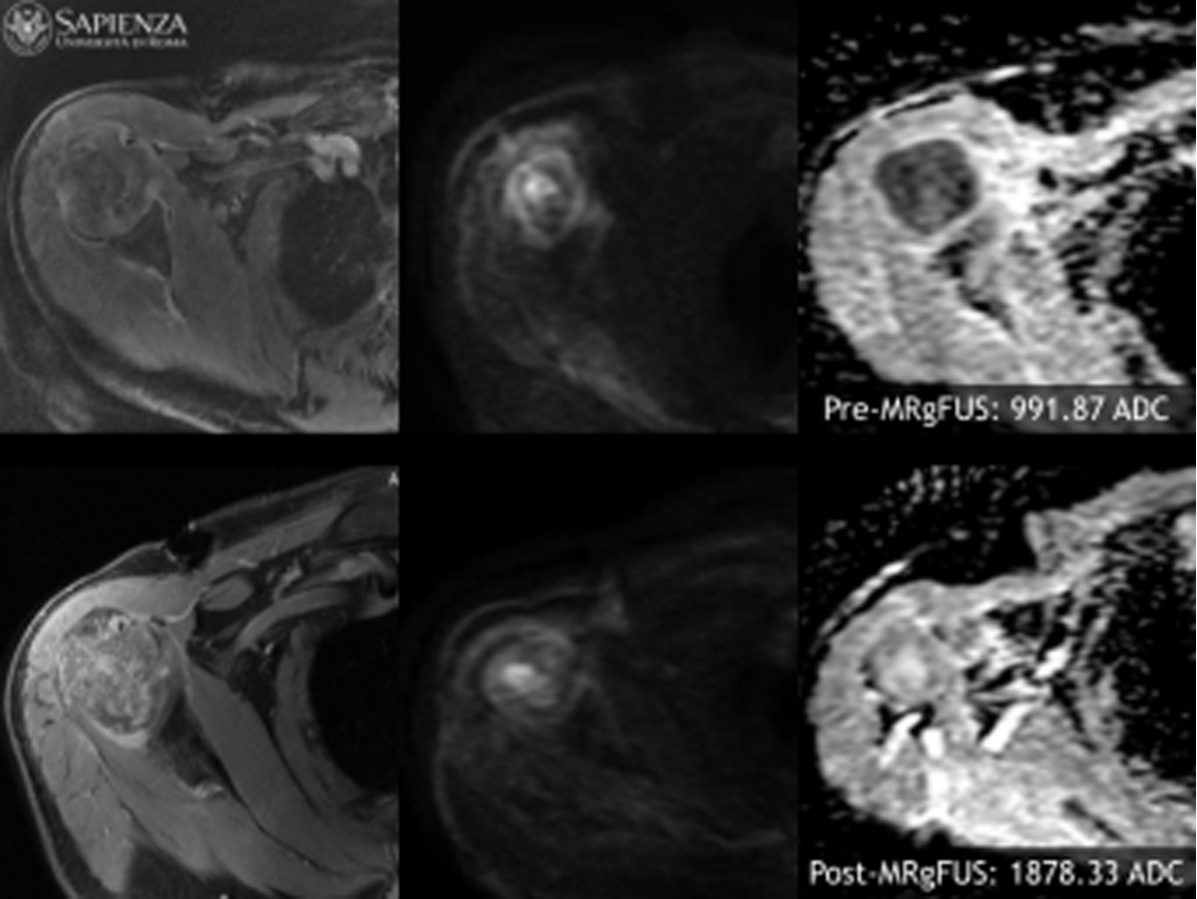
67 years old patient with lung cancer. MR images acquired before and after MR guided ultrasound treatment shows increase ADC value at 3 months follow-up (1878.33 mm^2^/s) compared to baseline (991.87 mm^2^/s). Also at the end of follow-up we observed absence of pain (VAS baseline: 7; VAS 3 months: 0).
